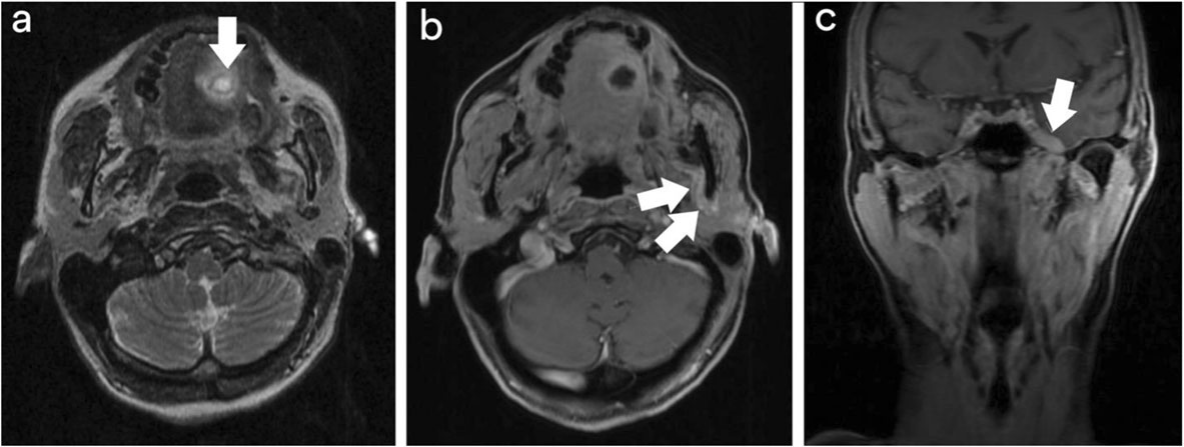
Representative MR neck images post-incisional biopsy. a T2-weighted axial MR sequence demonstrating the location of the primary lesion (arrow). b T1-weighted axial MR sequence post-gadolinium administration demonstrating asymmetric thickening posteromedial to the left ramus of mandible (arrows), corresponding to an area of anastomosis between cranial nerves V and VII. c T1-weighted coronal MR sequence post-gadolinium administration demonstrating asymmetric thickening along Meckel cave (arrow)
